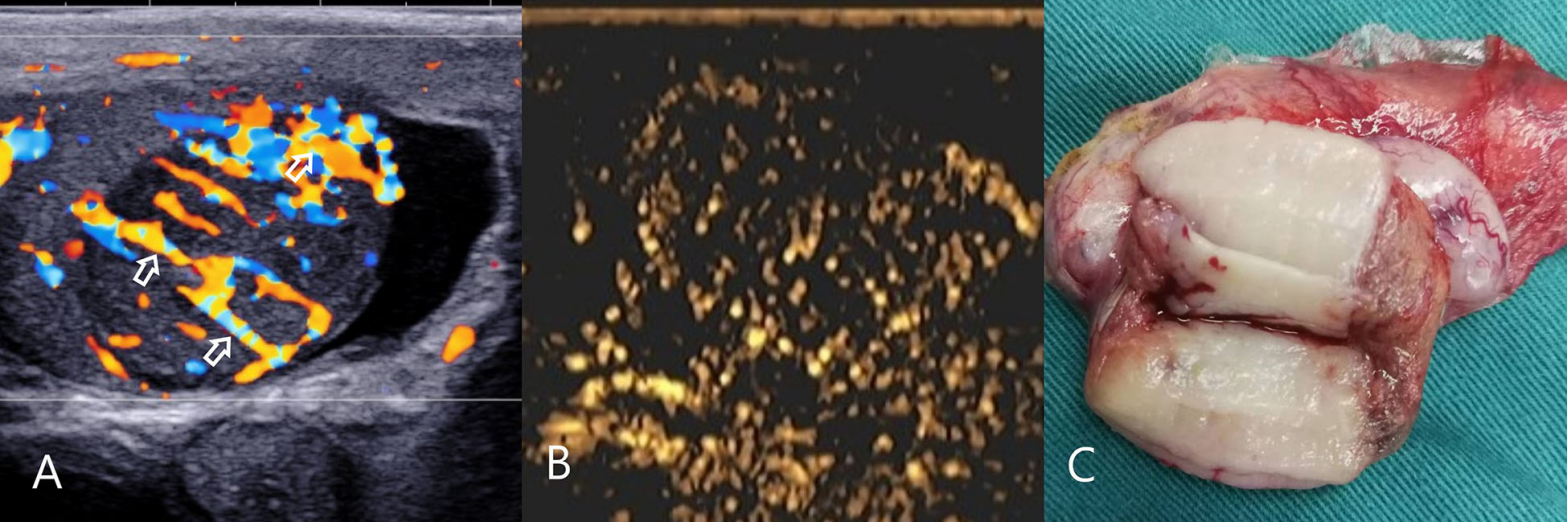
Testicular spermatocytic tumor. (A) CDFI: hypoechoic mass in the right testis, abundant blood flow and multiple perforating vessels(arrow), consistent with the ultrasound findings of lymphoma. (B) CEUS: The CEUS showed heterogeneous sparse and low enhancement, which was significantly different from that of lymphoma. (C) Macro-section, Mass occupies most of the testicle; CDFI, Color Doppler Flow Imaging; CEUS, contrast-enhanced ultrasonography.
